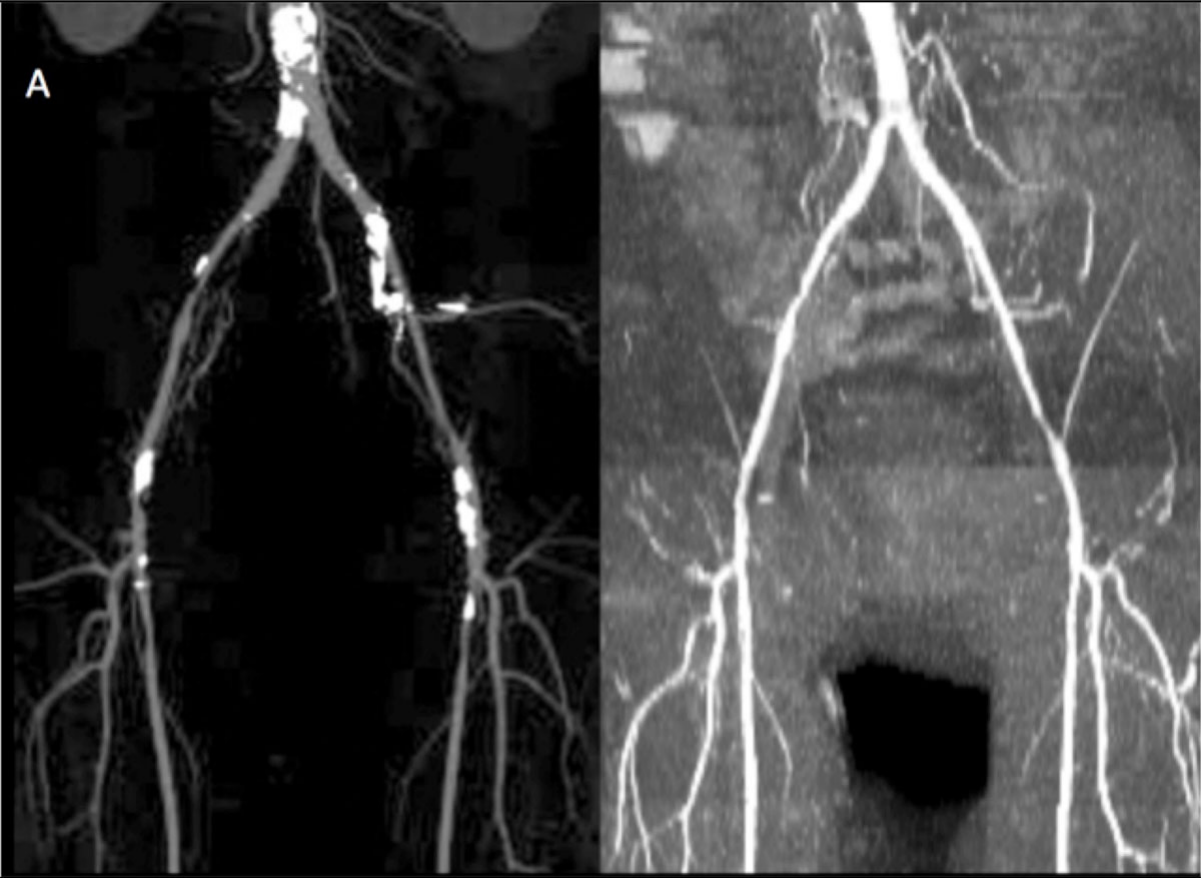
60-year old male with PAD. (A) MIP from a peripheral CTA (left) shows extensive vascular calcifications that partially obscure the arterial lumen, but are invisible in the MIP of the QISS MRA (right).
